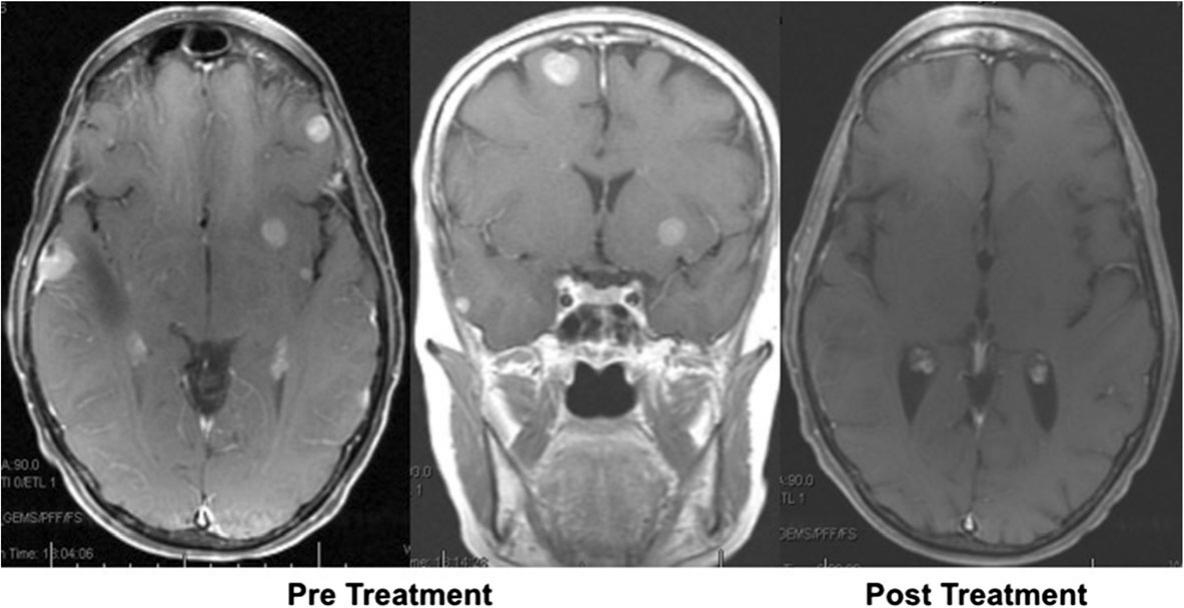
Pre- and post-treatment MRI findings showing near complete response to combination therapy with pembrolizumab and tocilizumab. Notably, the patient initially had 18 intracranial metastases that were completely resolved
